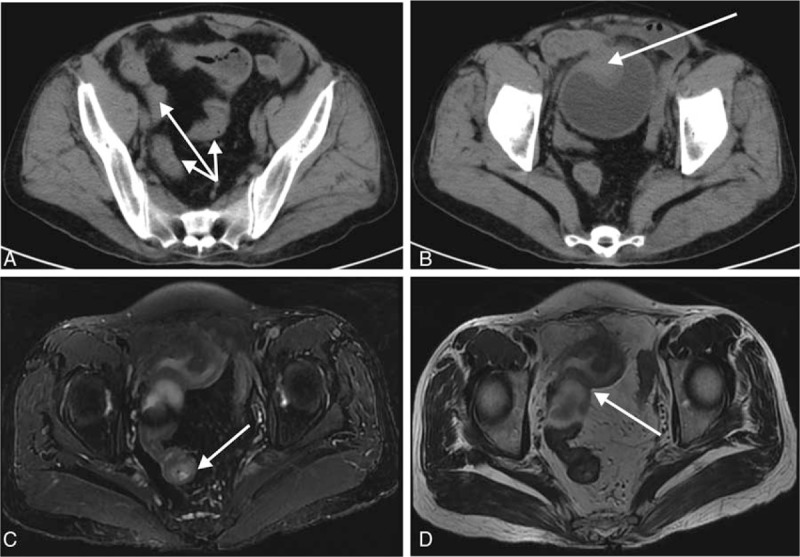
Computed tomography (CT) and magnetic resonance imaging (MRI) scanning images of a 58-year-old female with complicated Crohn disease. This patient presented with abdominal pain for >10 years, with a 3-month history of cloudy urine. Enhanced CT scanning showed a nidus with wall edema and mucosal thickness in the far end of the ileum (arrows in A), and the nidus invaded the bladder and shaped an eminence lesion, with a size of 38 × 23 mm (B). MRI scanning showed a mass convex to the enteric cavity of the ileum, with tresis and internal fistula (T2-weighted, C), and this inflammatory mass encroached on the anterosuperior bladder (T1-weighted, D).
